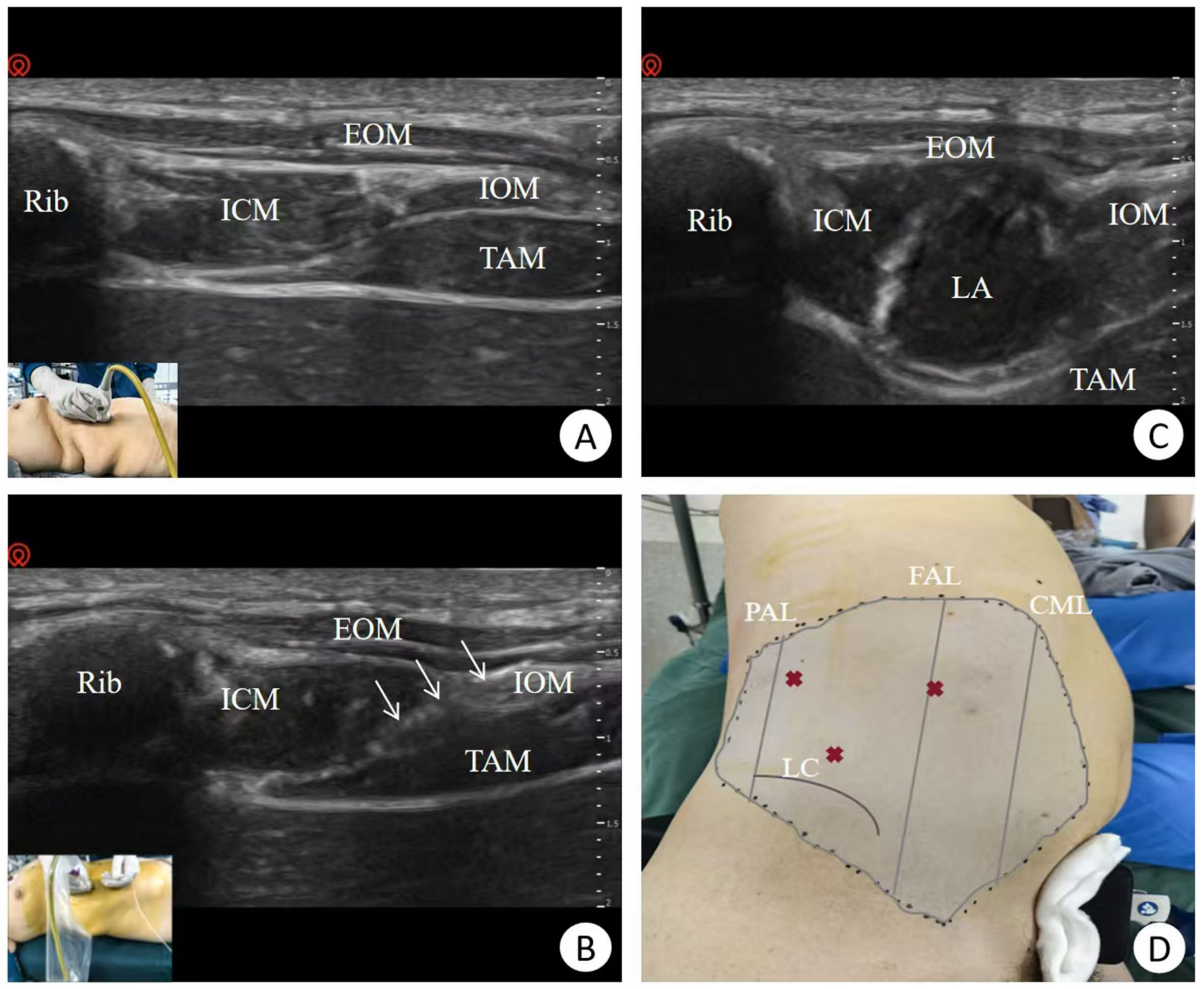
Ultrasound-guided M-TAPA via the perichondrial approach. (A) Ultrasound probe placement on the anterior axillary line showing the 10th rib and surrounding structures. (B) Ultrasound-guided nerve block puncture, the arrow indicates the visualization of needle. (C) Injection of local anesthetic solution. (D) Assessment of the nerve block range. PAL, Posterior axillary line; FAL, Front axillary line; CML, Clavicle midline; LC, Iliac crest; Rib, 10th rib; EOM, External oblique muscle; IOM, Internal oblique muscle; TAM, Transversus abdominis muscle; ICM, Intercostal muscle; LA, Local anesthetic.
